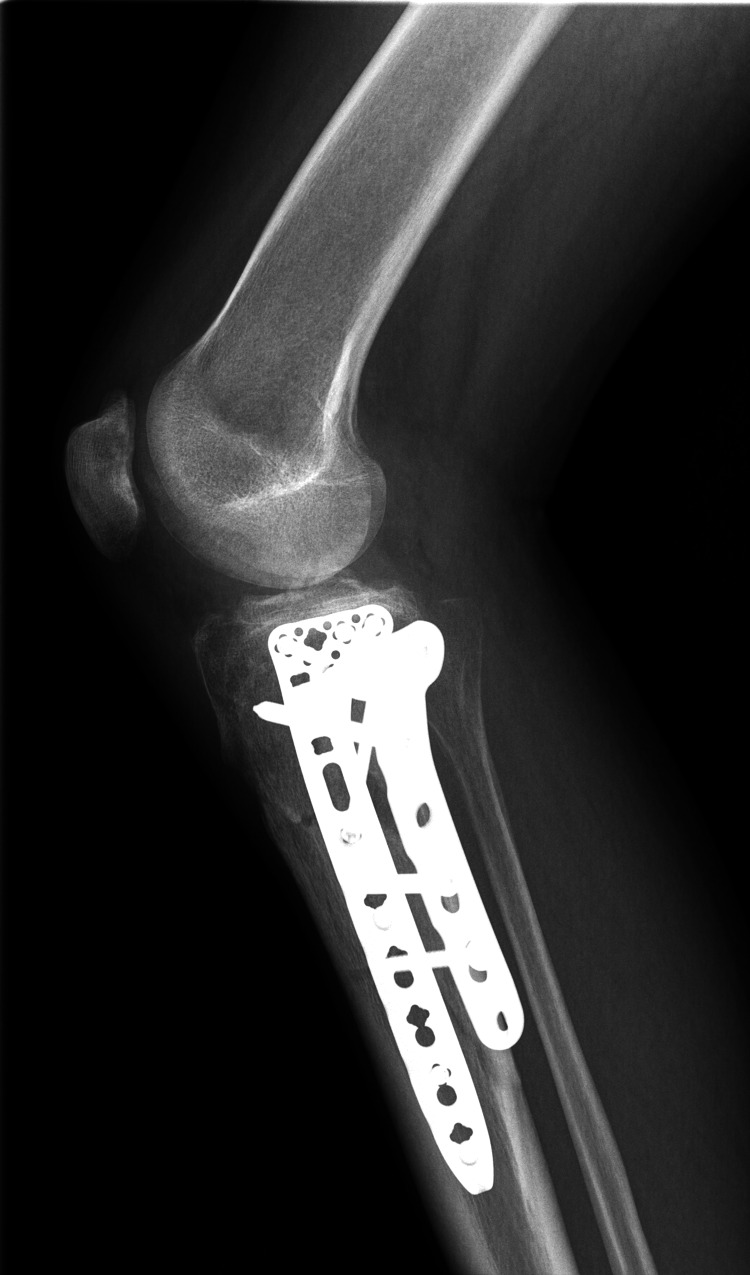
X-ray (Lateral view) showing the fracture after surgical intervention
